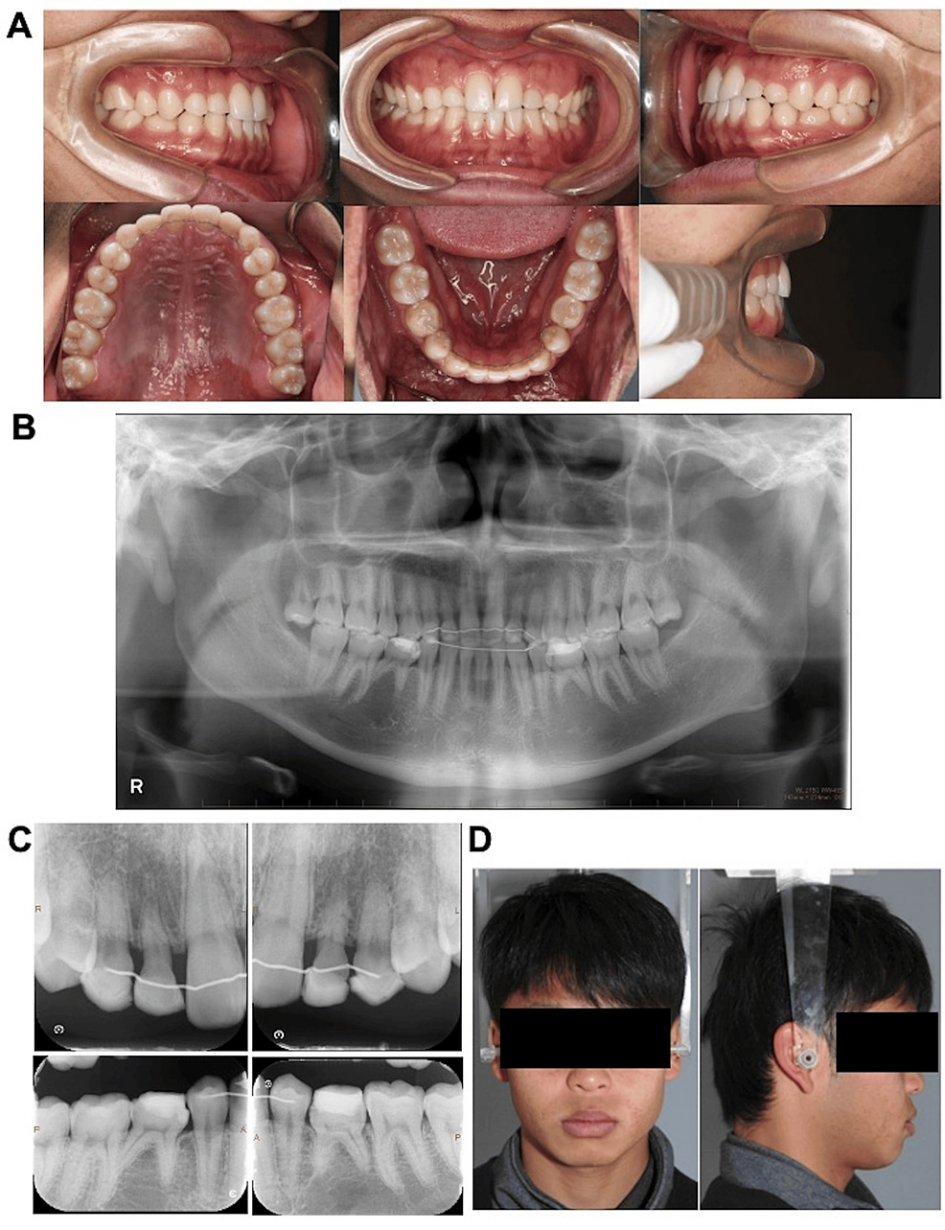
Two years after the start of the retention treatment A: intraoral photographs, B: panoramic radiograph, C: dental radiograph, D: facial photographs
